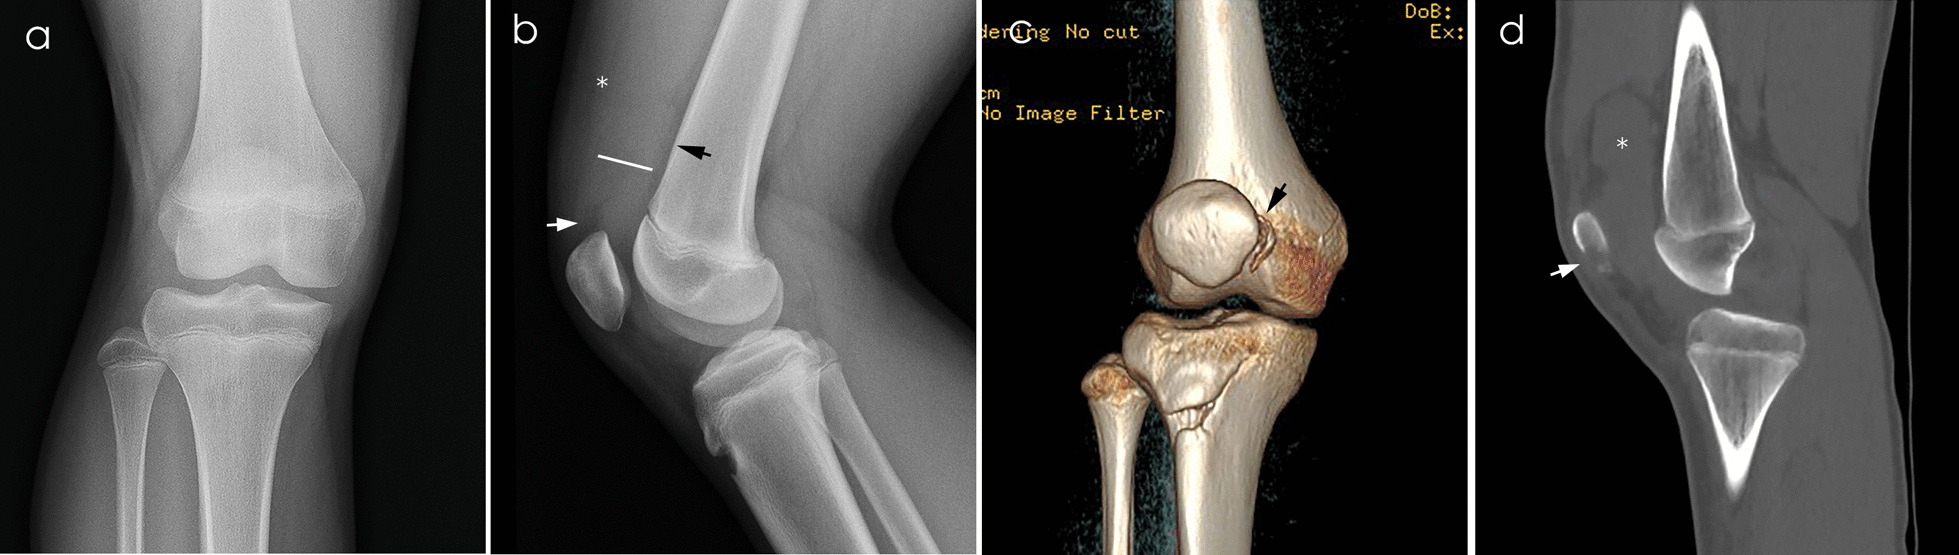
Plain radiograph and CT of a 13-year-old boy with right traumatic patellar dislocation. a Anteroposterior knee radiograph. b Lateral knee radiograph. The quadriceps fat (white arrowhead) and prefemoral fat (black arrowhead) are deep to the quadriceps tendon (star). SP effusion was measured with a fluid stripe (white line) intervening between the prefemoral fat and quadriceps fat. c CT after reconstruction showing fracture fragment at the medial inferior edge of the patella (black arrowhead). d Sagittal view of CT showing fracture line (white line) and obvious SP pouch effusion (star)
